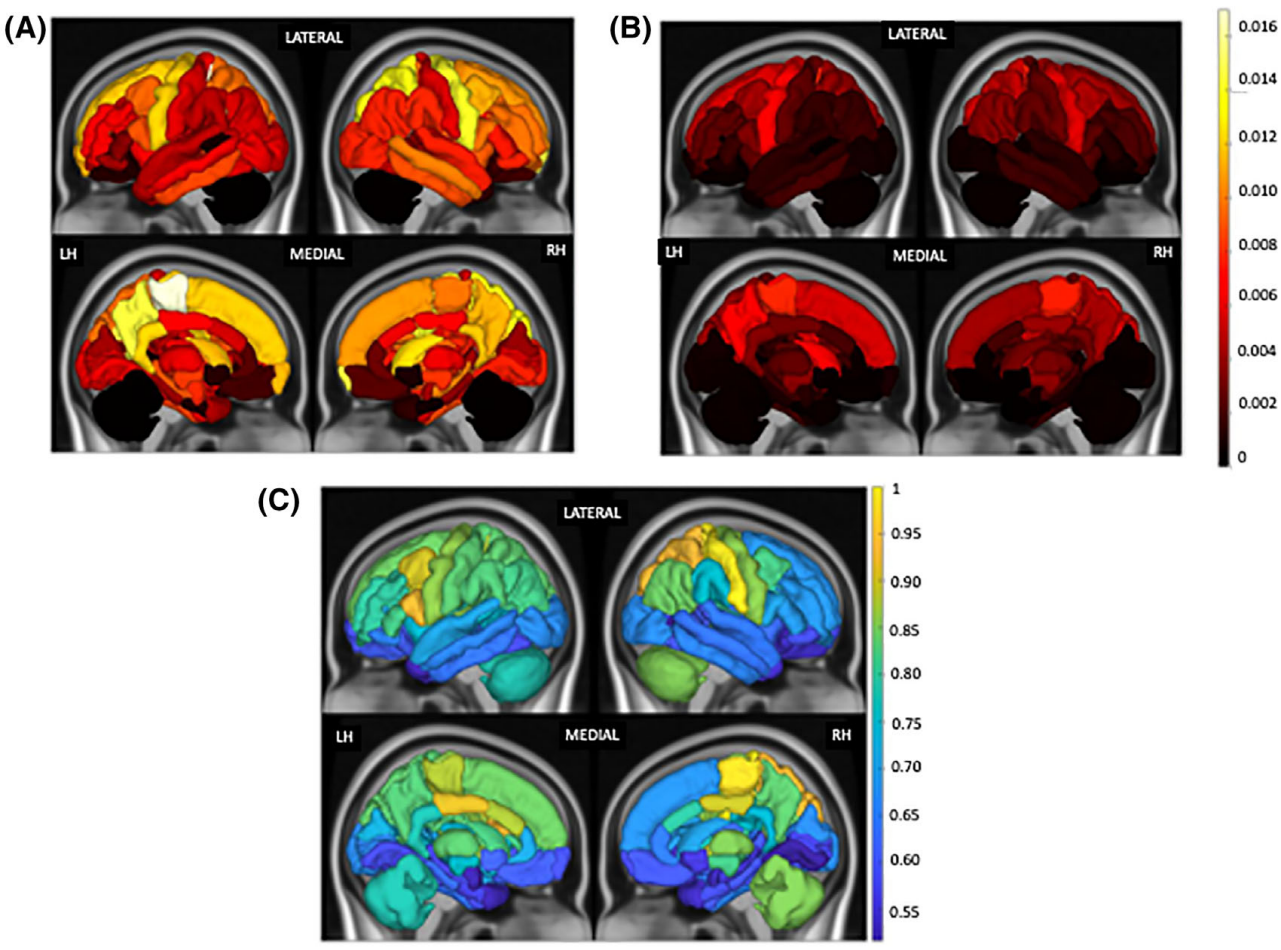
Median of ChaCo extracted from (A) rim‐ lesion mask (*T*2 FLAIR lesions excluding rim+ lesions) across all pwMS (N = 96) and (B) rim+ lesion masks, only for the pwMS who had at least one rim+ lesion (N = 56). The colorbar shows the ChaCo for the figure (A) and (B). (C) Relative paired Wilcoxon rank‐sum statistic (divided by maximum value) indicating all regions had greater ChaCo from rim‐ lesion masks than from rim+ lesion masks (considering only the 56 pwMS who had at least one rim+ lesion). Median ChaCo from rim+ lesion masks were computed only for the subjects who had rim+ lesions (N = 56), while the median ChaCo from the rim‐ lesion mask was computed across all subjects. Note the scale differences in the two modalities—this is mostly due to the fact that there were far fewer rim+ lesions than rim‐ lesions
